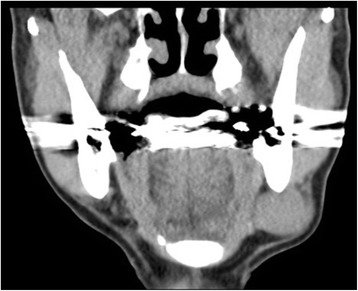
Computed tomography imaging of the tumor. A well-demarcated tumor is located in the left submandibular region (coronal plane)
